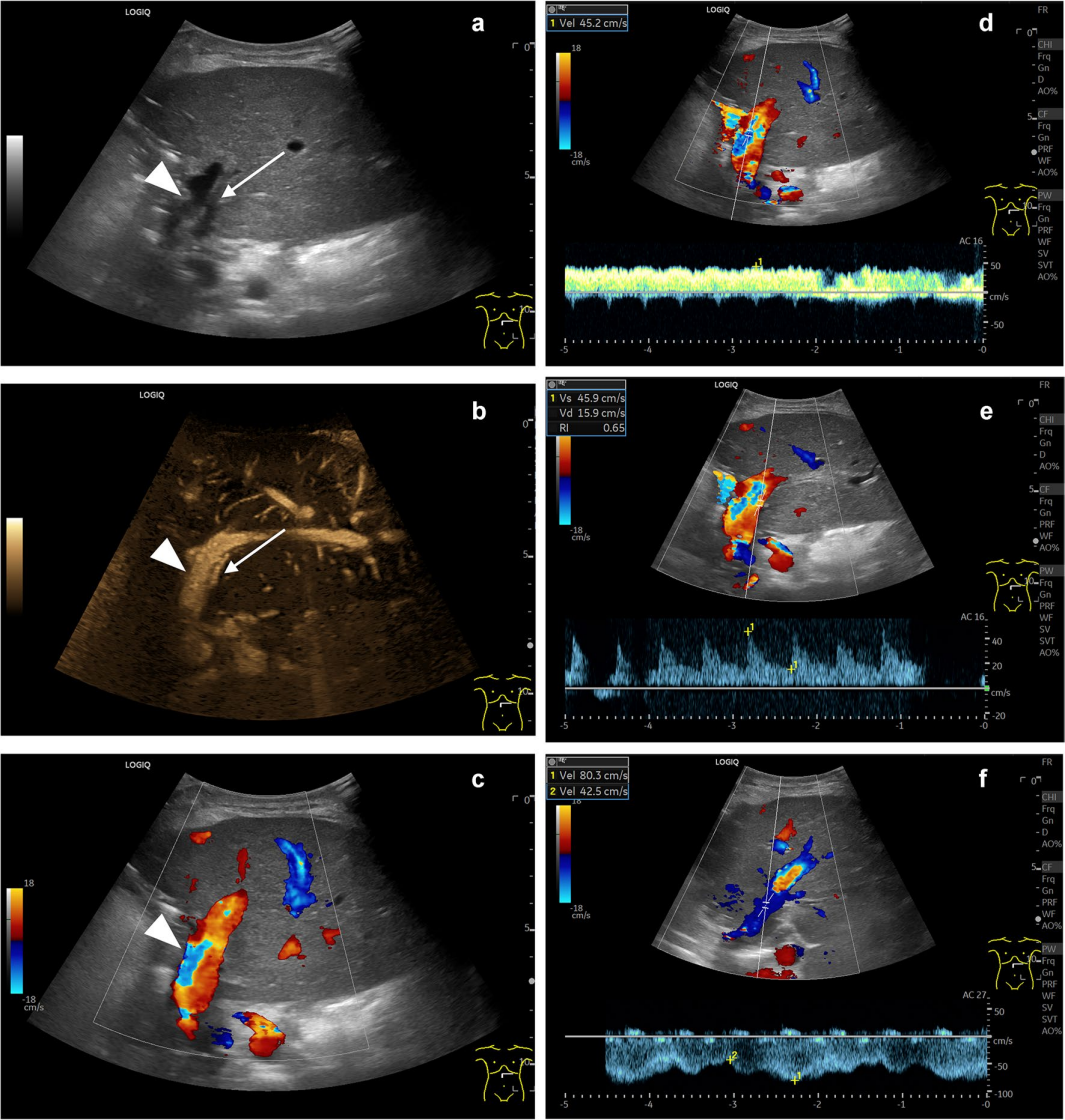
Postoperative ultrasound in transverse plane on day 21 after combined kidney and liver transplantation in an 8-year-old girl with a diagnosis of methylmalonic acidemia. The left-lateral split liver transplant (segments 2, 3) is shown in the upper abdomen. On B-mode (a) and B-flow (b), the neighboring portal vein (*arrowheads *in a and b) and hepatic artery (*arrows *in a and b) can be seen entering the neo-hilum. On the color Doppler image (c), the hepatic artery is not distinguishable from the portal vein as the color signal extends beyond the vessel’s true boundaries (blooming artifact). Regular flow patterns are noted in the portal vein (d), hepatic artery (e), and hepatic vein (f)
